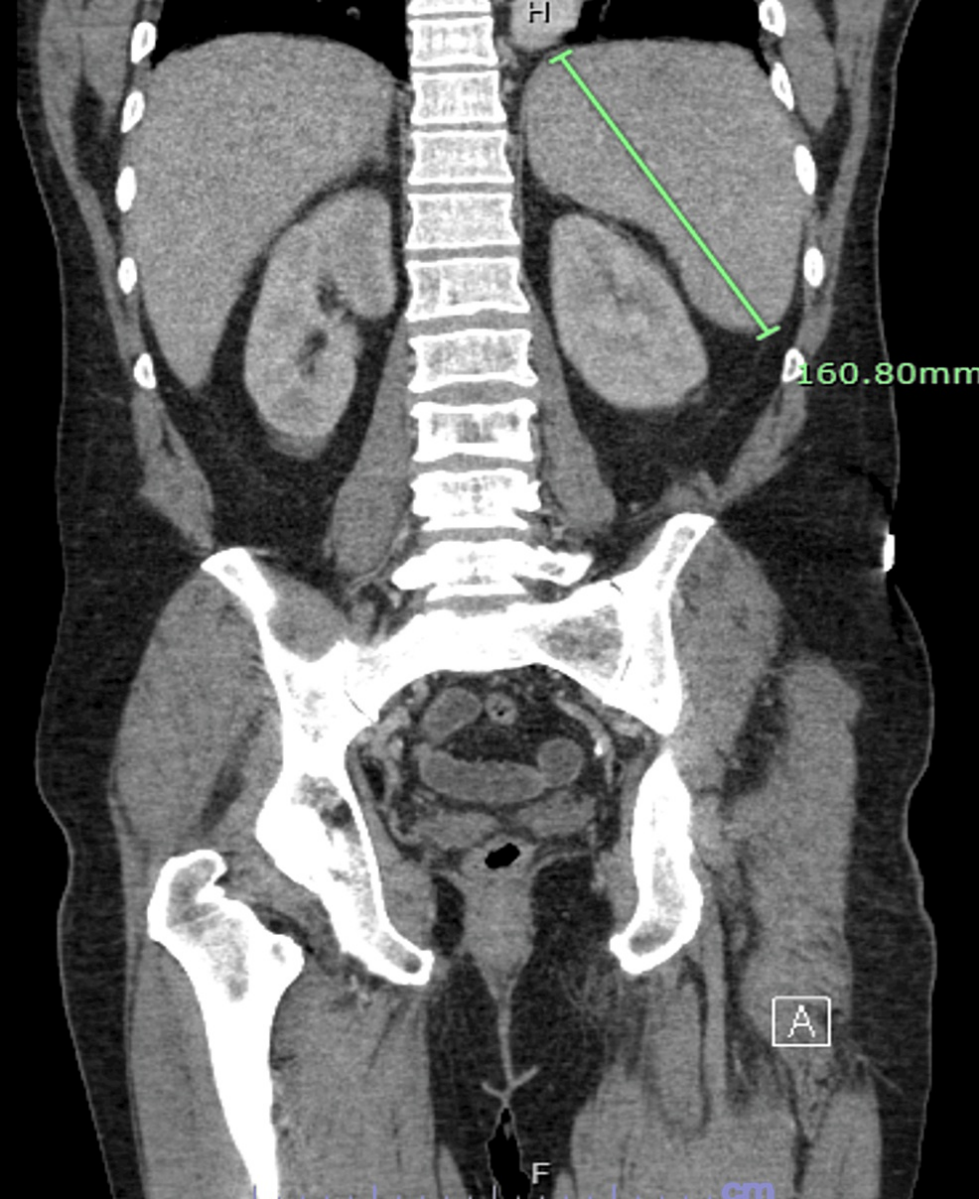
Cross section of contrast-enhanced CT scan showing splenomegaly, measuring approximately 16 cm
